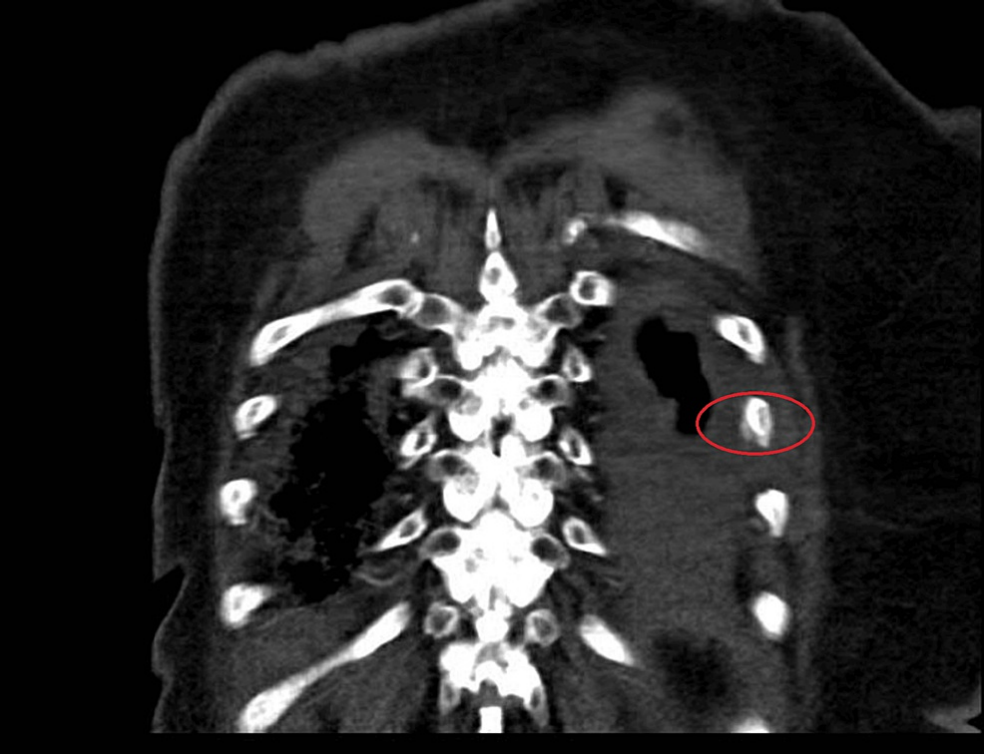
High-resolution axial CT image showing cortical disruption of left lateral rib 8.
